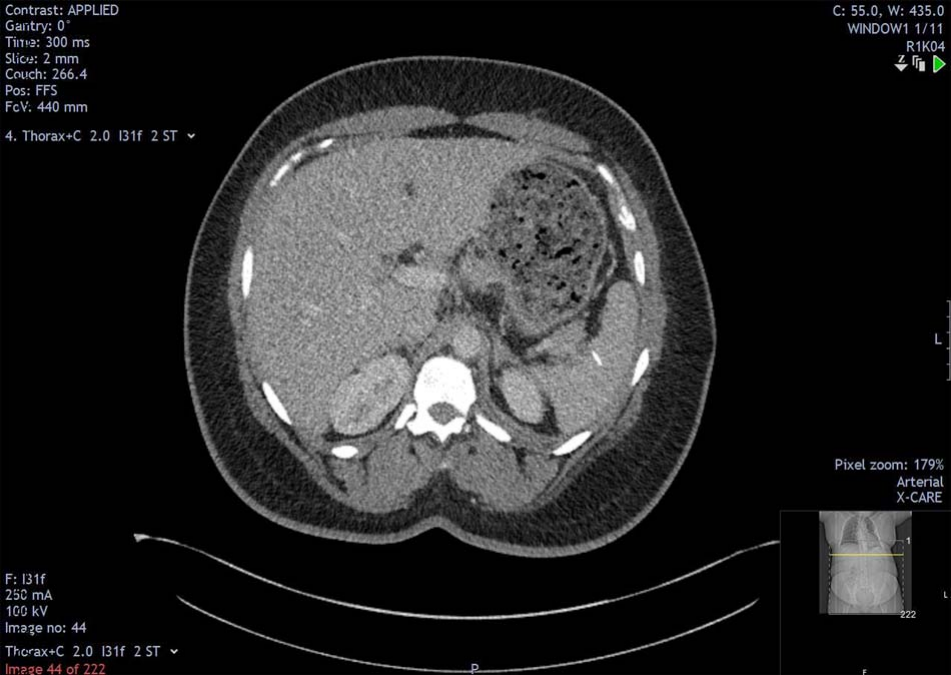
Two-mm axial section from the outpatient CT scan performed in November 2021, demonstrating resolution of the perisplenic haematoma with foreign body *in situ.*
